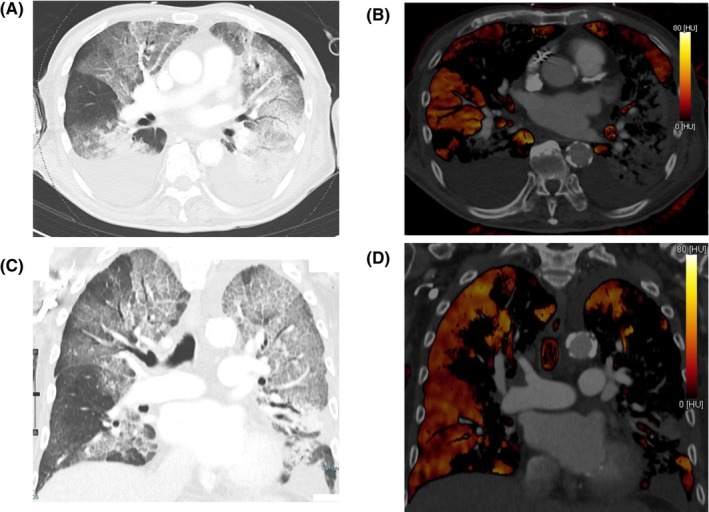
Plain CT and dual‐energy CT image at the presentation (Day 1). (A) Plain CT image of the transverse plane. (B) Dual‐energy CT image of the transverse plane. (C) Plain CT image of the coronal plane. (D) Dual‐energy CT image of the coronal plane. (A–D) show perfusion defects consistent with pulmonary infiltrates in both lungs.
